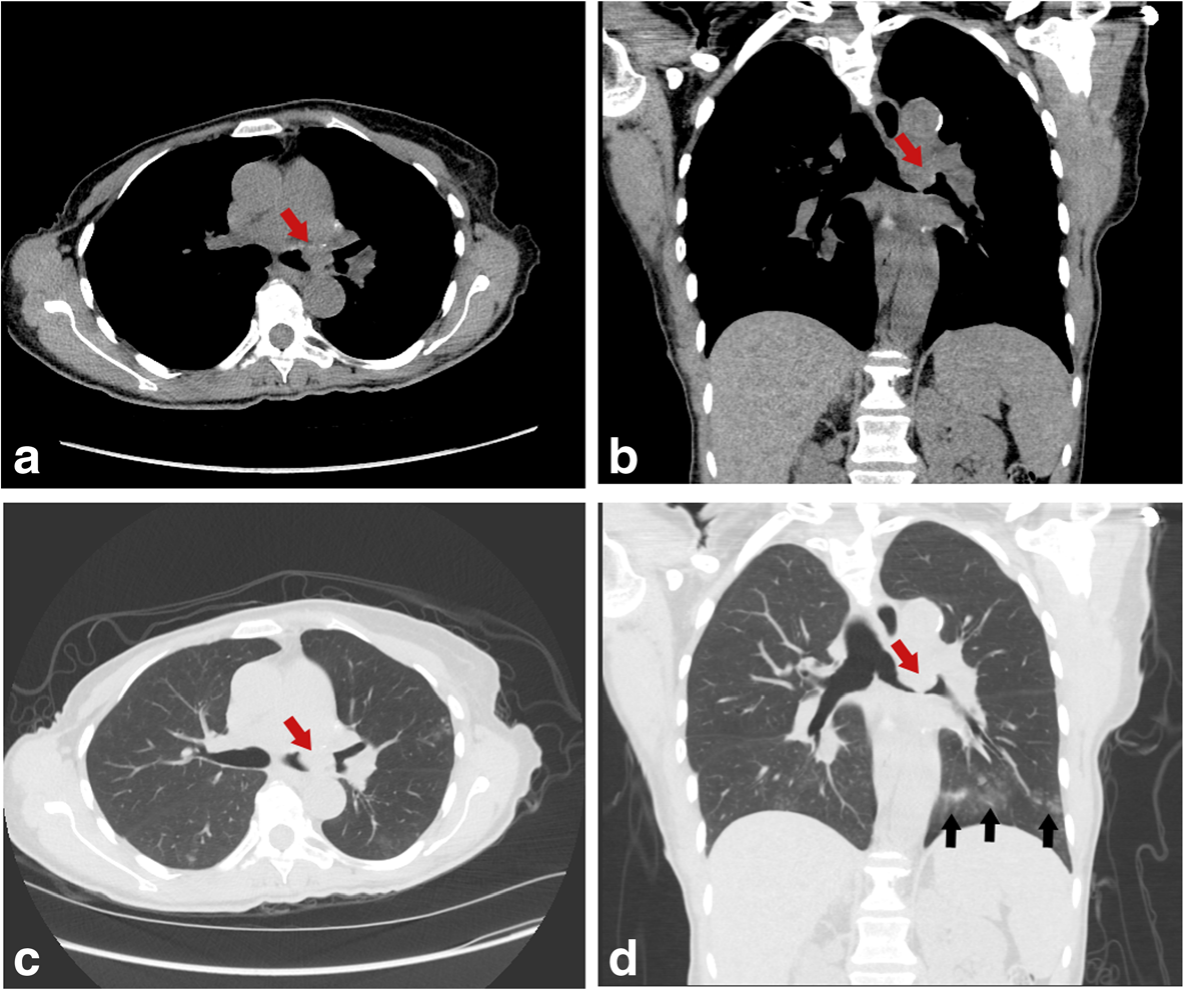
Representative computed tomography axial a and coronal b mediastinal windows which demonstrate a 2.2×1.1×0.9 cm polypoid soft tissue lesion projecting from the roof of the left mainstem bronchus (*red arrow*) adjacent to the bronchial anastomotic site. There is severe narrowing of the left mainstem bronchus with the residual lumen measuring approximately 3 mm. Computed tomography axial c and coronal d lung windows demonstrate diffusely decreased attenuation of the left lung parenchyma, compatible with diffuse air trapping secondary to obstructive endobronchial lesion. There is lower lobe predominant tree-in-bud nodularity (*black arrows*)
